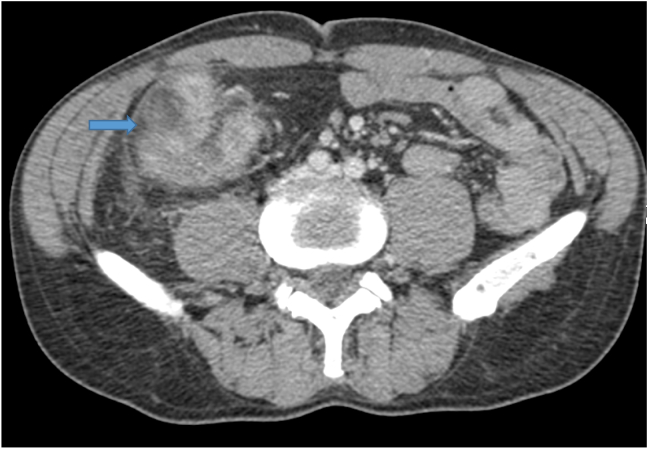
Abdominal CT Scan in the axial plane revealing the intestinal mass.
